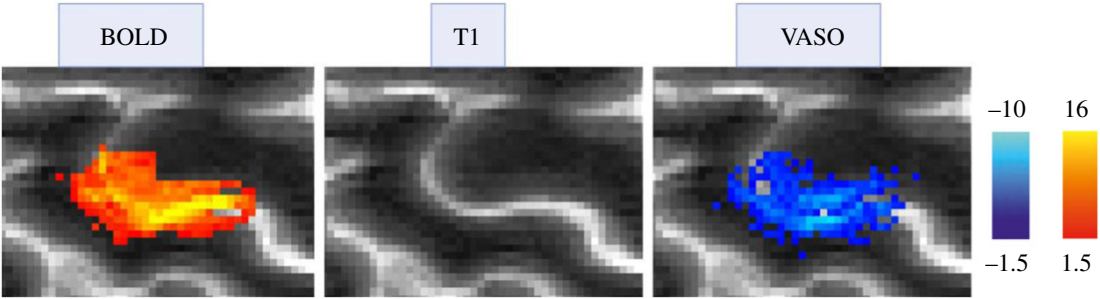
Functional MRI using BOLD contrast and cerebral blood volume maps acquired with slab-selective vascular occupancy imaging (VASO). Comparison of single-subject activation maps generated by finger tapping, in an axial section through the central sulcus. Echo-planar data acquisition, resolution 0.74 × 0.74 × 2 mm^3^, TE = 20 ms, TR = 1.5 s (interleaved VASO and BOLD acquisition; figure courtesy of Laurentius Huber).
